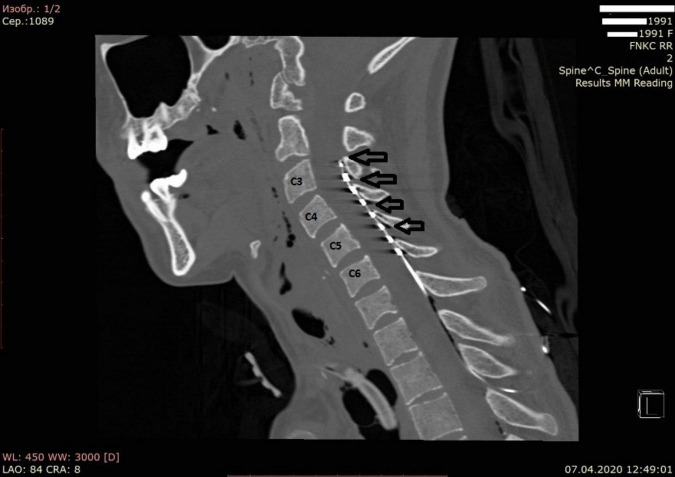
Computed tomography of the cervical spine in bone mode in the sagittal projection. The arrows indicate the position of the electrodes.
